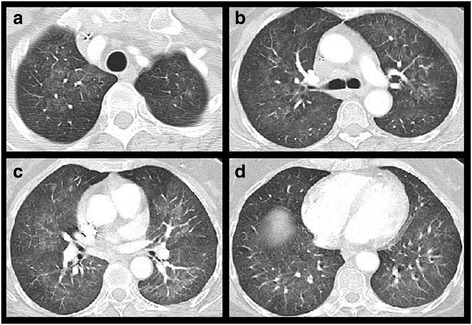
Pneumocystis jirovecii pneumonia. Patient with adjuvant chemotherapy for breast cancer. Chest CT at the level of the lung apex (a), tracheal bifurcation (b), apical segments of the lower lobes (c) and the dome of the right diaphragm (d) at lung windows: Diffuse, symmetrical, bilateral ground glass sparing the lung periphery both in the axial plane as well as lung apices and bases
